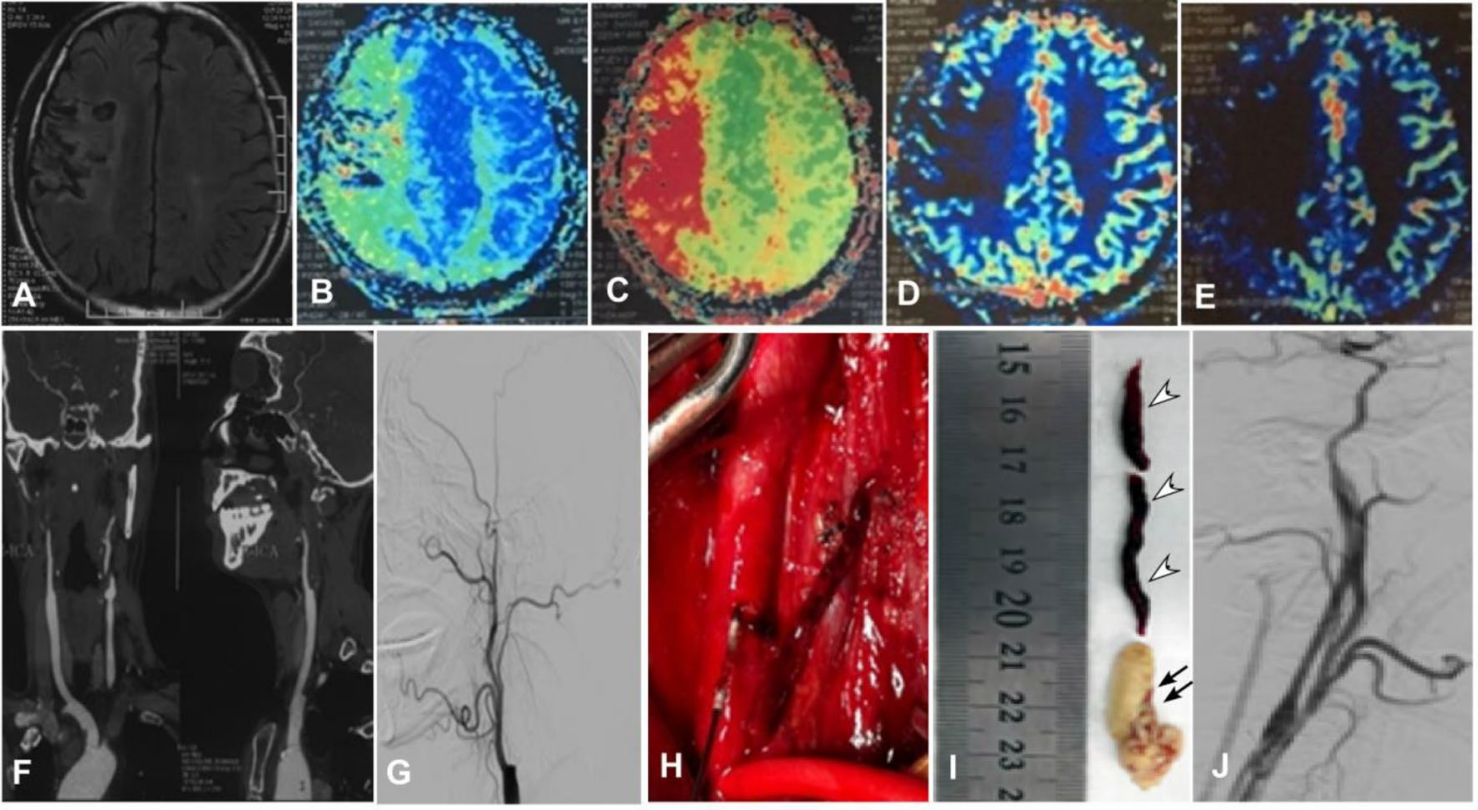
Proximal atherosclerotic plaques involving the common carotid artery bifurcation and causing long-segment occlusion of the right internal carotid artery (ICA) treated with hybrid surgery comprising carotid endarterectomy (CEA) and endovascular treatment in a patient in their 50 s. (A) Magnetic resonance imaging (MRI) showed multiple infarcts in the right semioval region. (B–E) The time-to-peak (B) and mean transit time (C) were prolonged, and the cerebral blood volume (D) and cerebral blood flow (E) were decreased. (F) Computed tomography angiography revealed occlusion of the right ICA. (G) Digital subtraction angiography demonstrated occlusion of the right ICA. (H) CEA was conducted, and a Forgarty balloon was used for removal of the atherosclerotic plaque. (I) The atherosclerotic plaque (double arrows) and the thrombi (arrow heads) were approximately 8 cm long. (J) After the surgery, the ICA occlusion was successfully recanalized.
